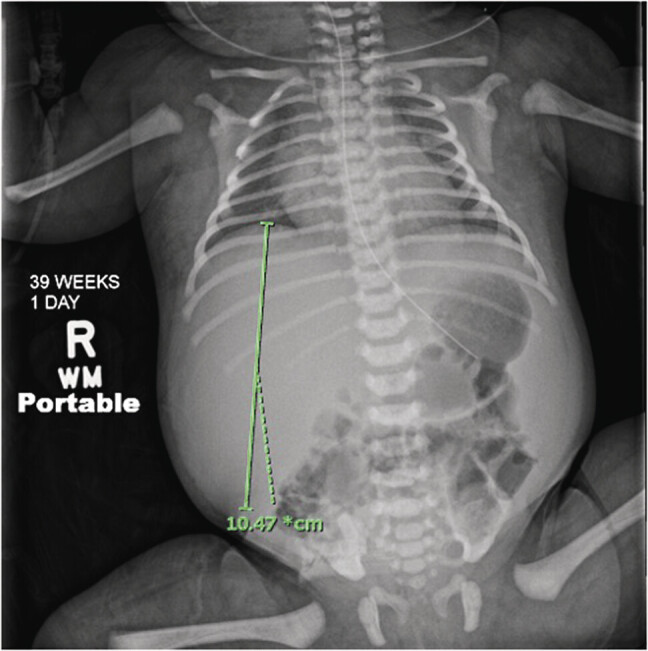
Abdominal X-ray image taken on DOL 0 showing liver at 10.5 cm in the mid-axillary line and splenic enlargement.
